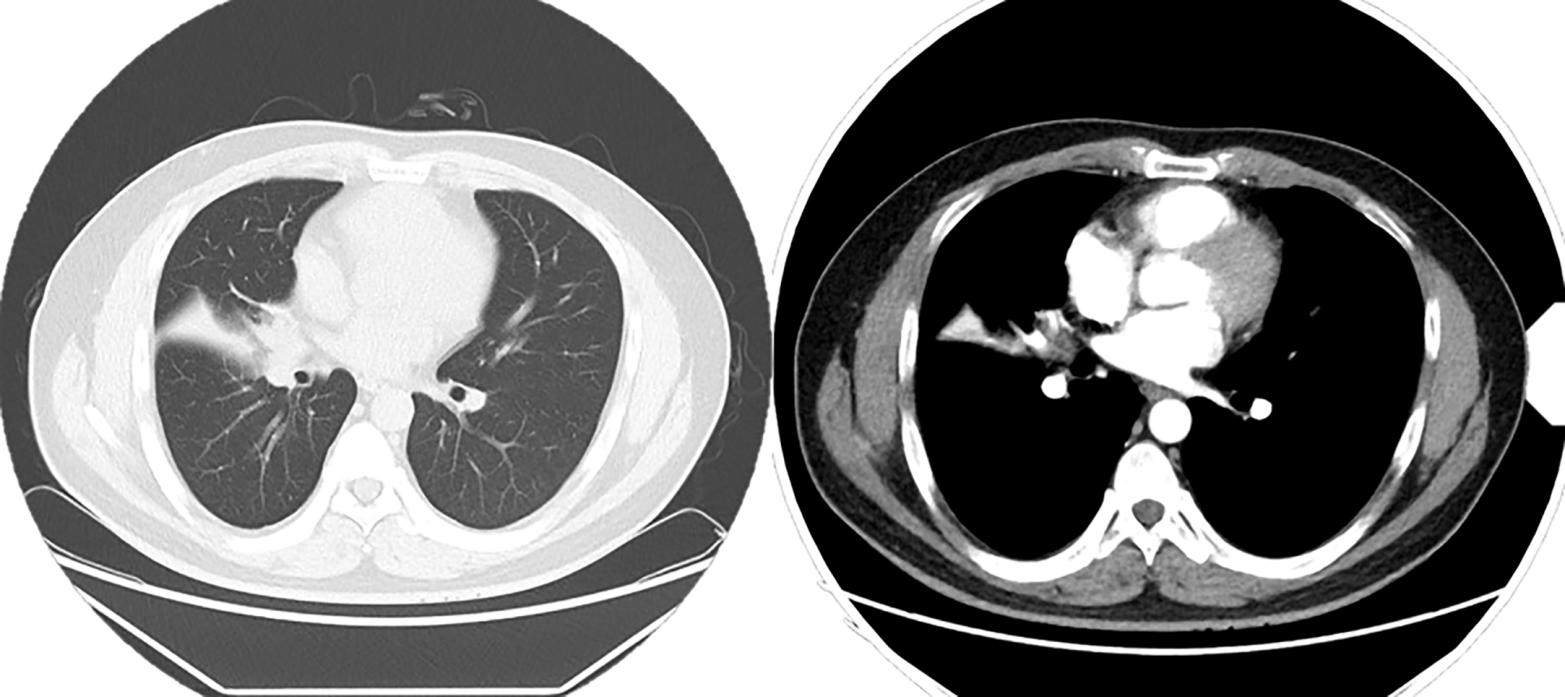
Chest CT enhancement scan: Soft tissue nodule is seen at the right hilum, measuring approximately 2.0 × 1.7 cm, and mild enhancement is seen on the right lung middle lobe atelectasis, with a patchy hyperdense shadow and right lung middle lobe bronchus occlusion. A ground-glass nodule shadow is seen in the dorsal segment of the lower lobe of the right lung, measuring 7 × 5 mm, approximately anteriorly. An enlarged lymph node shadow is seen in the right hilum, approximately 1.0 cm in diameter.
